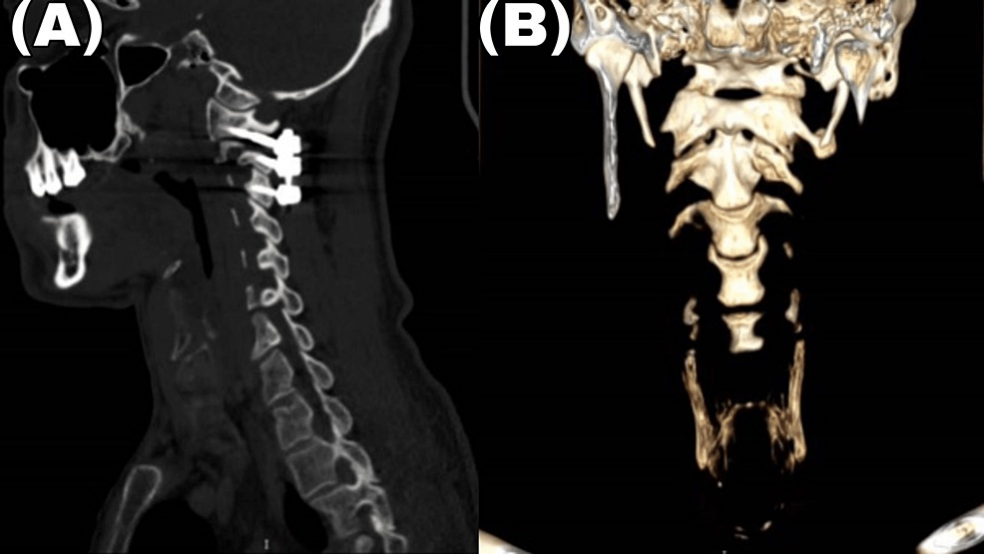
Postoperative CT of the cervical spine Sagittal (a) and CT with 3D (b). Bone window CT of the cervical spine after 10 months of surgery demonstrates a reduction of the locked facet and good alignment of C1 and C2.
